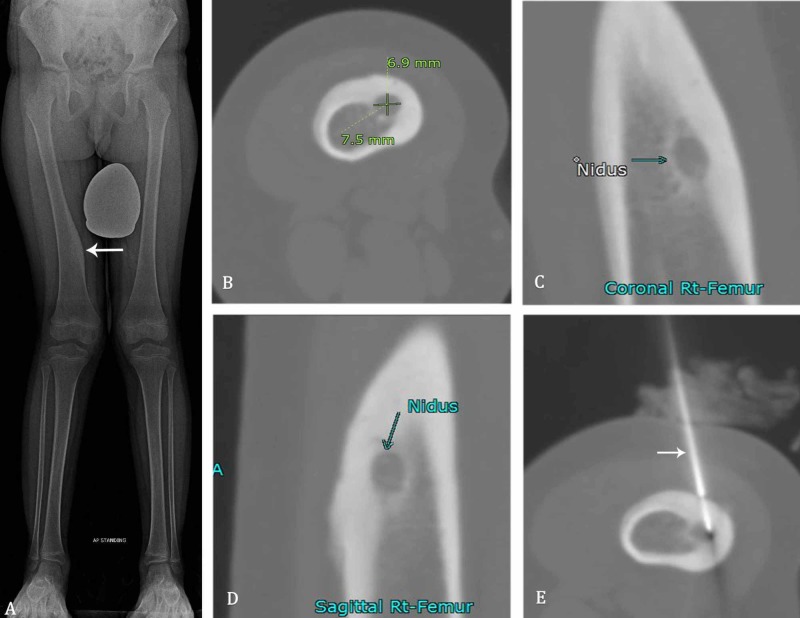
Various imaging findings of a six-year-old boy with an osteoid osteoma along the medial margin of the right femoral diaphysis. A) Scanogram radiograph showing osteoid osteoma in the medial right femur with associated cortical thickening (arrow). B-D) Nidus (arrows) is measured and well-demarcated on unenhanced CT-extremity images with surrounding cortical thickening. E) Soloist needle (arrow) is within the nidus and used for radiofrequency ablation.
